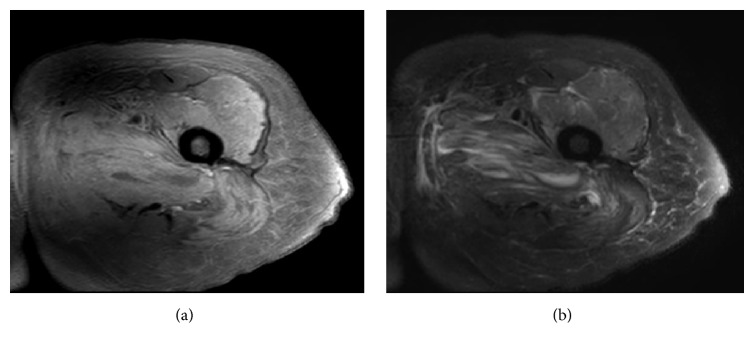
(a) MRI axial T1 with gadolinium, (b) axial T2 without gadolinium showed collection sized 2.9 × 3 cm with intramuscular edema.
